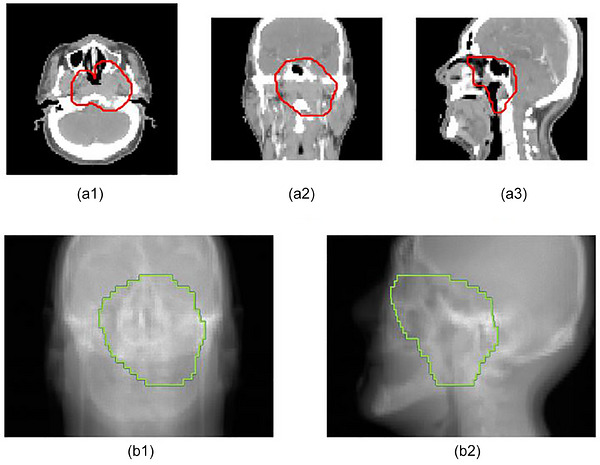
Example of MLC‐based dynamic collimation for an NPC patient. (a1–a3) Axial, coronal, and sagittal CT images of the patient with the corresponding VOI delineation. The red contours indicate the VOI used for conformal imaging. (b1, b2) Representative CT forward projections at different projection angles with the corresponding MLC‐defined aperture. The green contours indicate the projected shape of the MLC aperture on the detector plane.
